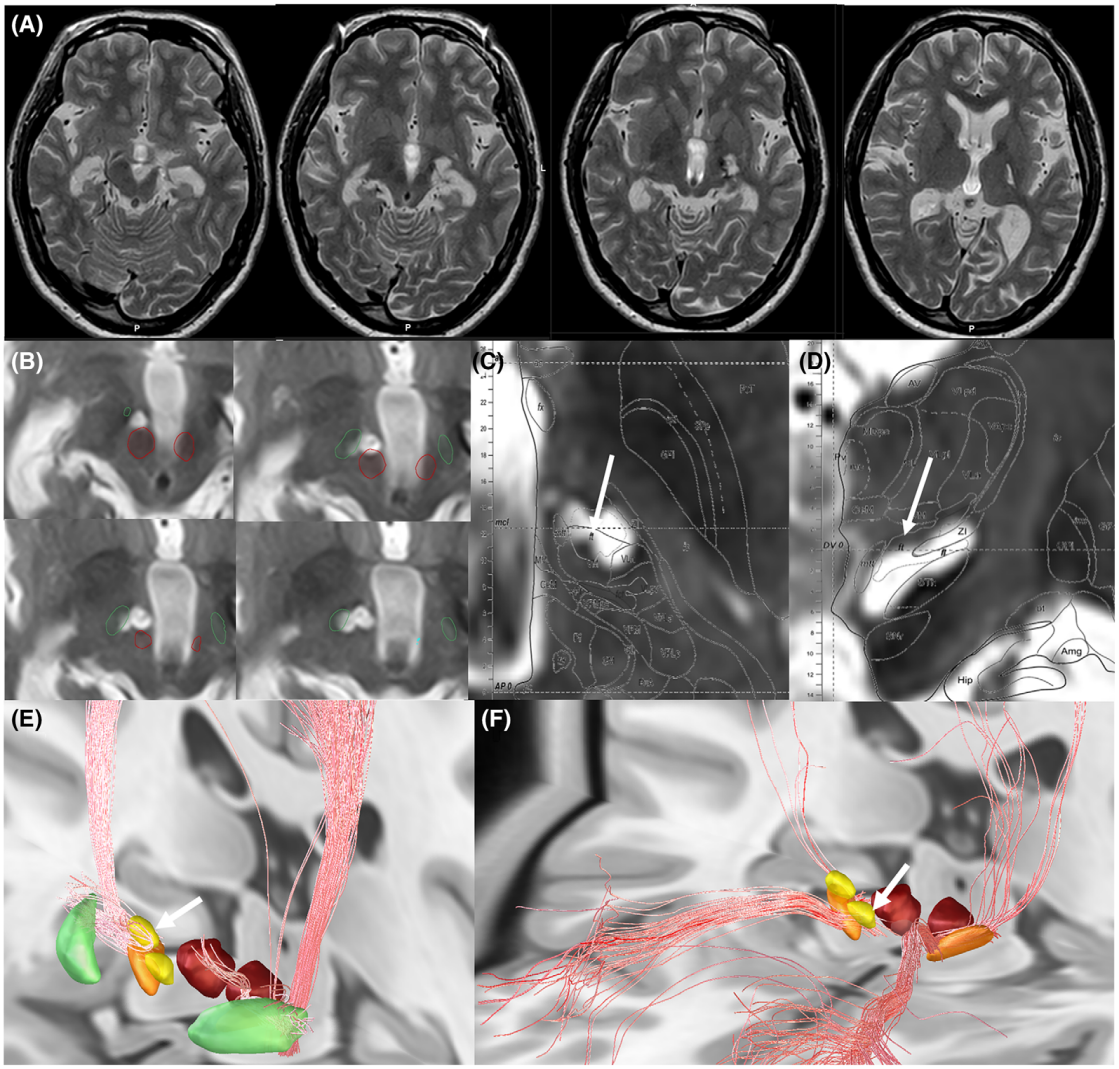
Postoperative imaging analysis. (A) Preoperative axial T2‐weighted MRI showing post‐traumatic lesions in the left hemisphere. (B) 3‐month postoperative T2‐weighted axial MRI showing a medial lesion anterior to the red nucleus and a lateral lesion supero‐medial to the subthalamic nucleus. Green: subthalamic nucleus; red: red nucleus. (C and D) Anatomical location of the pallidothalamic tract at the fields of Forel shown by postoperative axial (C) and coronal (D) left–right flipped MRI overlaying Morel's atlas. White arrow represents thalamic fasciculus (ft) covered by the radiofrequency lesions. The area of the ft is called by fields of Forel H1. (E) Fiber tracts showing optimal stimulation network for cervical dystonia by Lead‐DBS. Lateral lesion (white arrow) is on the fiber tracts. Green: globus pallidus internus; orange: subthalamic nucleus; crimson: red nucleus; yellow: lesions. (F) Fiber tracts showing effective network for obsessive–compulsive disorder by Lead‐DBS. Medial lesion (white arrow) is on the fiber tracts. Orange: subthalamic nucleus; crimson: red nucleus; yellow: lesions.
